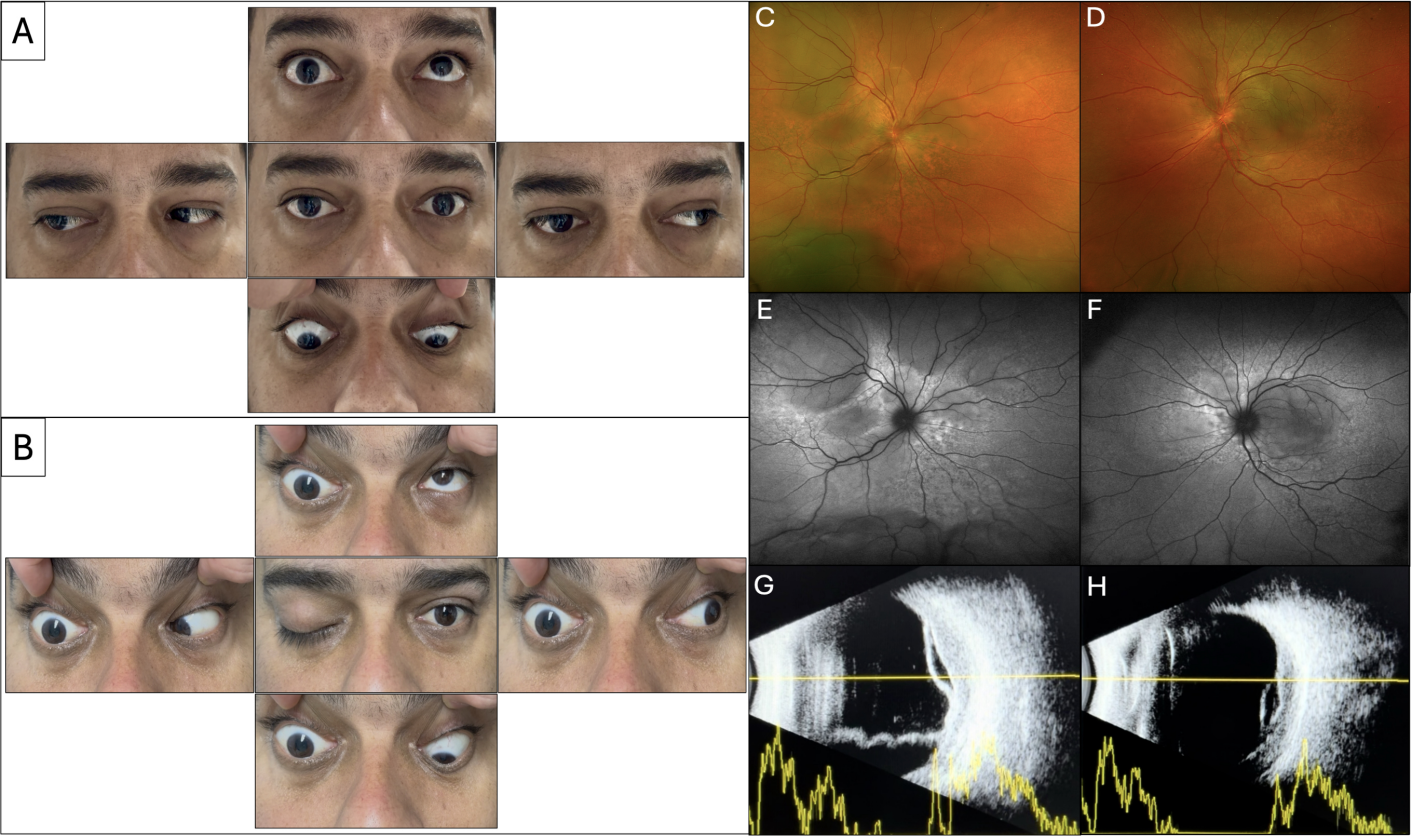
(A) Examination at presentation demonstrates limitation in extraocular movements in all directions of the right eye with associated mild ptosis. Movements in the left eye were complete in all gazes. No proptosis was noted. (B) Evaluation at the 3-day follow-up visit shows worsening ophthalmoplegia with progression to full ptosis on the right side. Extraocular movements in the left eye remain unchanged. (C, D) Fundus photographs demonstrate bilateral, multifocal SRD with subretinal fluid extension to the optic nerves, and patchy areas of hyper- and hypopigmentation in both the posterior pole and peripheral retina. (E, F) FAF revealed multifocal areas of hyper- and hypo-autofluorescence associated with SRD in both eyes. (G, H) B-scan ultrasonography confirmed the SRD and demonstrated areas of increased choroidal thickness. No evidence of posterior epibulbar extension or vitreous opacities was observed.
